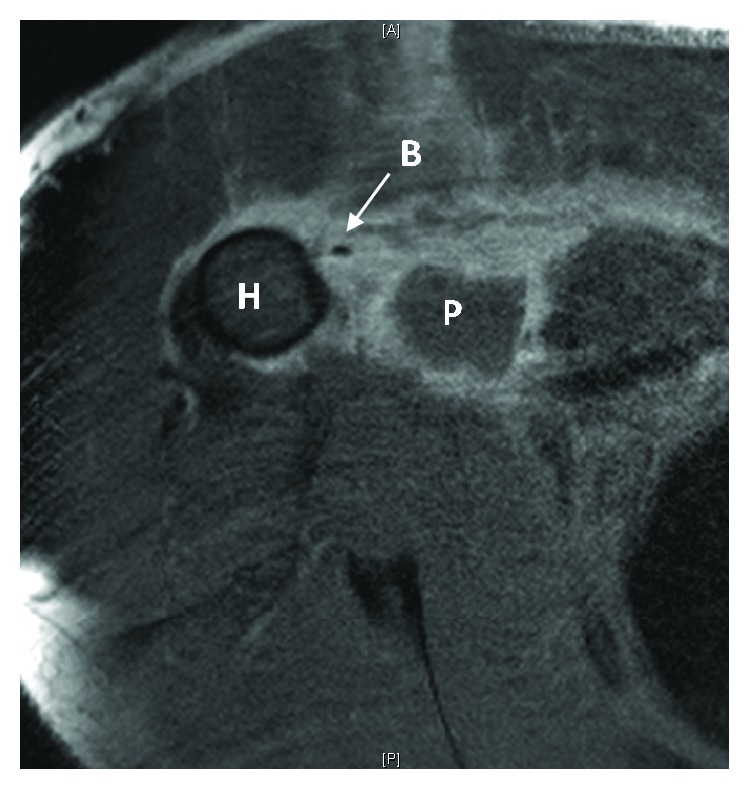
Axial MRI image. H: humerus; P: pectoralis major; B: biceps tendon.
